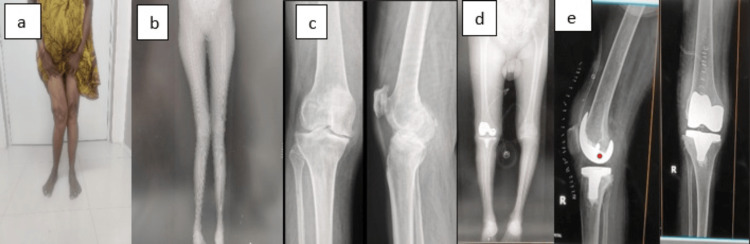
Case 1 (a) Preoperative clinical photograph. (b) Preoperative scanogram. (c) Preoperative radiograph. (d) Postoperative scanogram. (e) Postoperative radiograph.
